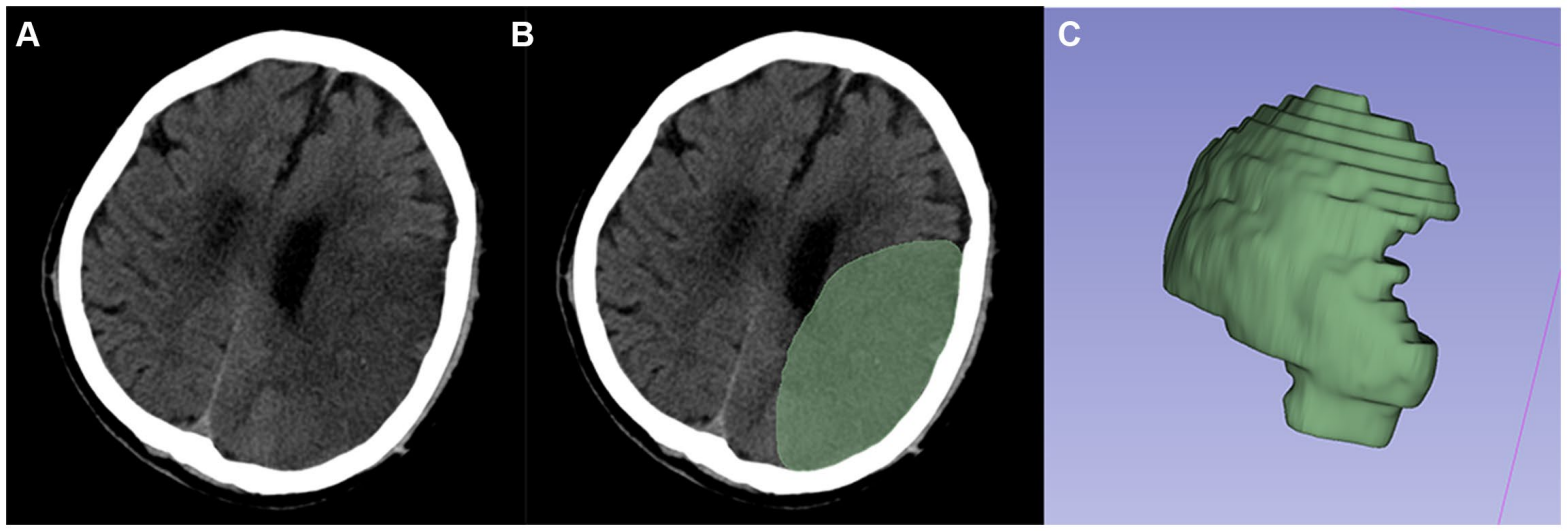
Typical example of ROI segmentation. (A,B) Segmented infarcted lesion (green area) on the axial slice of CT images. (C) 3D-ROI.
